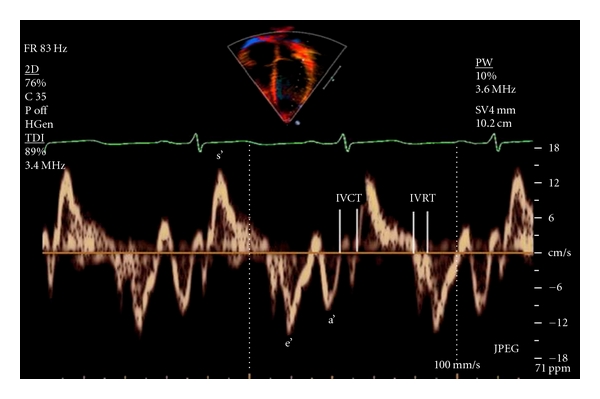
Lateral mitral annular tissue Doppler tracing. s′: peak velocity during ventricular systole; e′: peak velocity during early ventricular diastole; a′: peak velocity during atrial contraction; IVCT: isometric (isovolumic) contraction time; IVRT: isometric (isovolumic) relaxation time.
